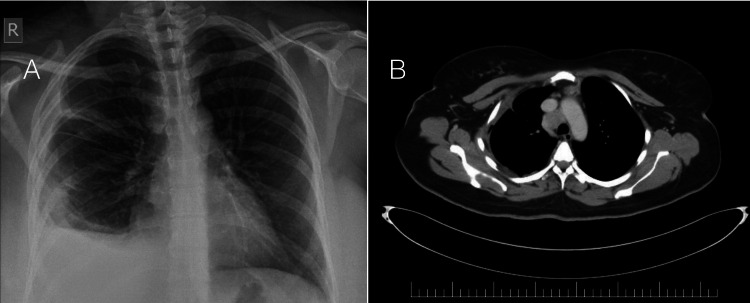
Patient's chest X-ray and computerised tomography Chest X-ray (image A) demonstrates obliterated right costophrenic angle, suggestive of pleural effusion. Computerised tomography (image B) of the chest revealed a large right paratracheal lymph node measured at 2.6 × 2.6 × 3.2 cm with central low attenuation suggestive of necrosis. R: right side.
